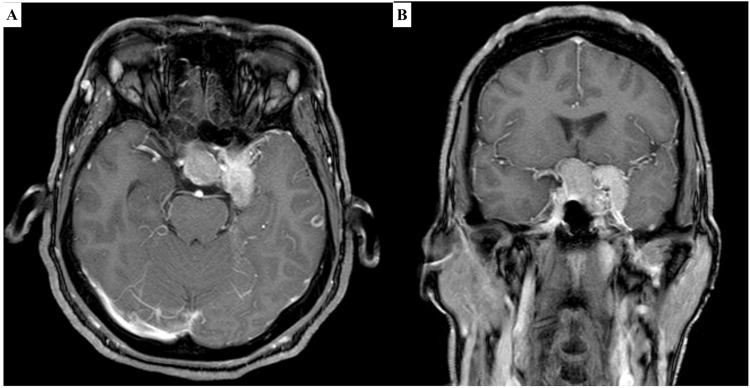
Preoperative magnetic resonance imaging The contrast-enhanced sagittal T1-weighted image (A) and coronal T1-weighted image (B) vividly depict an intrasellar mass, measuring approximately 19x19.8x21 mm with a subtle suprasellar bulge and homogeneous enhancement, suggestive of a Knosp 2 pituitary adenoma. Additionally, an adjacent left paresellar cavernous sinus-involving lesion is evident, measuring 16.5x23.5x26 mm. This lesion showcases a heightened contrast enhancement, prominently encasing the left internal carotid artery. The distinct characteristics observed raise the suspicion of a meningioma.
